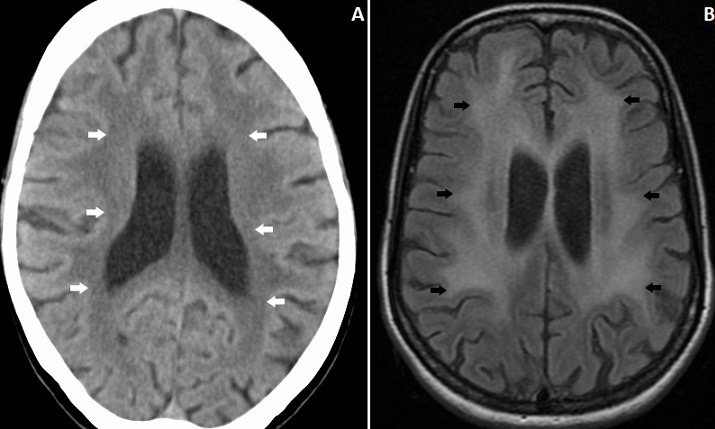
A) axial CT showing periventricular hypodensity (arrows); B) MRI Brain FLAIR image highlighting the periventricular lesions
